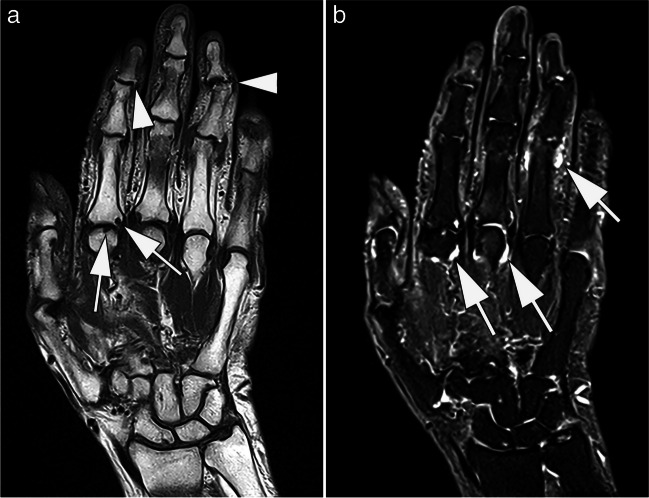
MRI at 0.55 T of the hand of a 77-year old patient with suspected late-onset rheumatoid arthritis. Coronal T1-weighted (A) image shows erosions at the 2nd metacarpophalangeal joint (arrows). In addition, osteophytes are depicted at the 2nd and 4th distal interphalangeal joints (arrowheads). B Corresponding STIR image shows synovitis at the metacarpophalangeal joints 2 and 3, and at the proximal interphalangeal joint 4 (arrows). TA: 03:20 min (A), 05:11 min (B)
